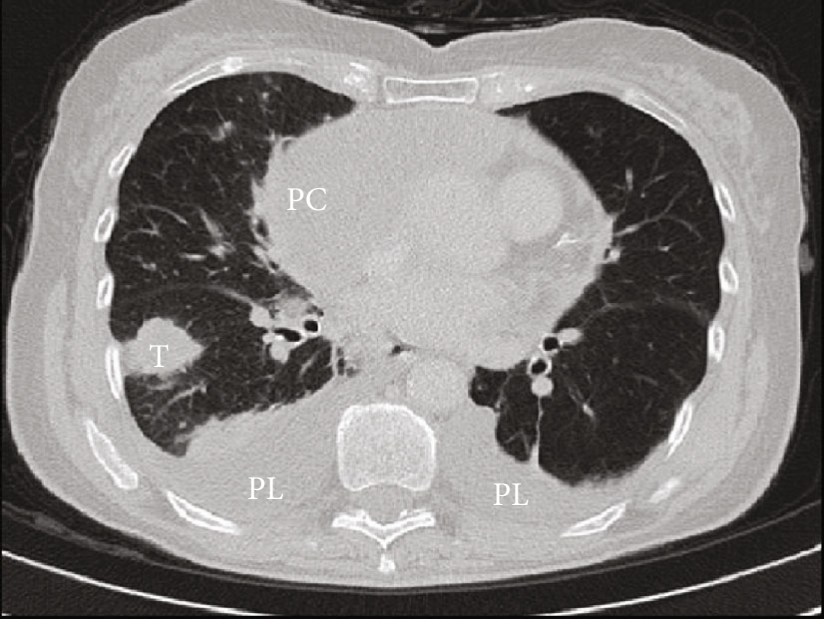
Computed tomography of the chest. PC—encapsulated pericardial effusion; PL—bilateral pleural effusion; T—tumoral mass in the right inferior lobe.
